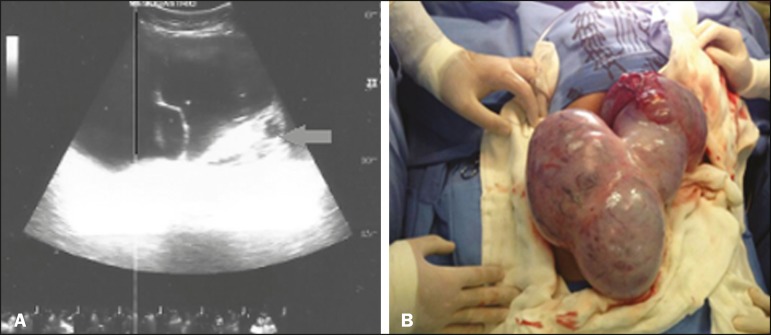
A: Ultrasound of the abdomen, showing a massive solid-cystic formation with a
